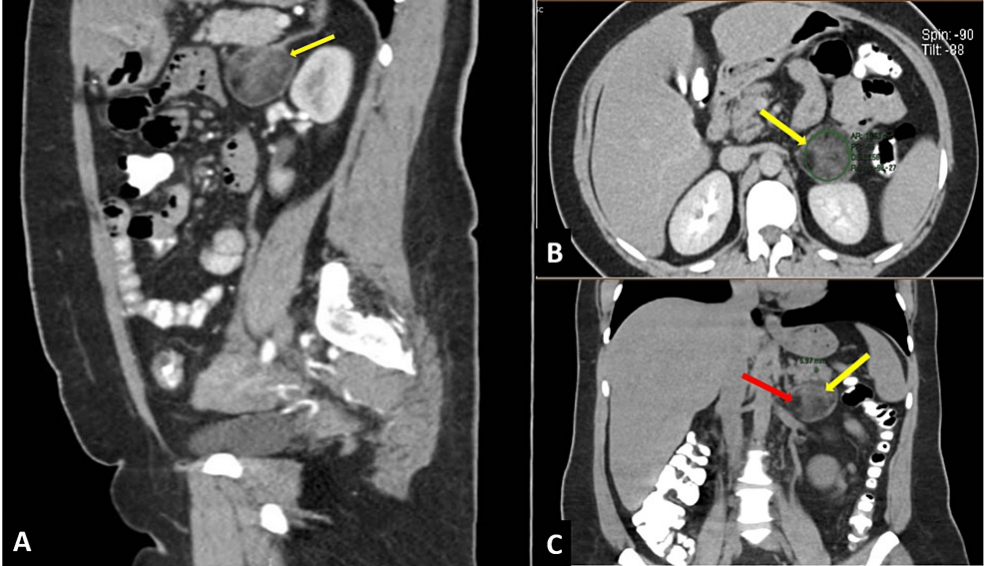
CT Scan of the Abdomen and Pelvis A) Sagittal section: The tumor (yellow arrow) maintains a separation plane with adjacent structures (small intestine, pancreas, left kidney, and adrenal gland). B) Axial section: A solid tumor (yellow arrow) with a thin and well-defined wall is observed, showing a heterogeneous pattern with areas of up to -60 Hounsfield Units (HU). C) Coronal section: The interior of the tumor is heterogeneous, with hypodense areas corresponding to adipose tissue (yellow arrow) and hyperdense areas corresponding to myeloid tissue (red arrow), measuring 4.3x4.6x4.7 cm in dimensions.
